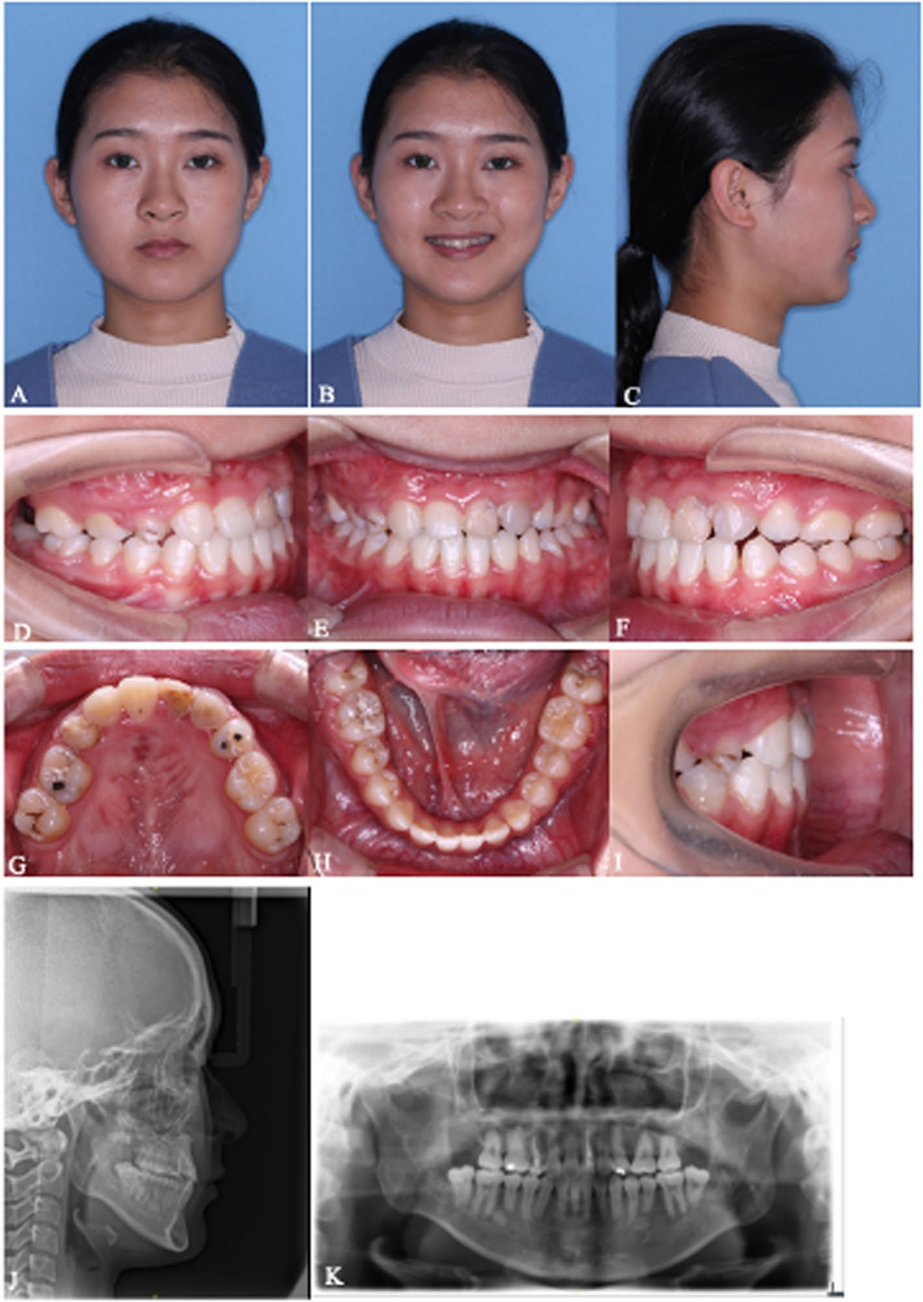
A-K After orthognathic surgery and postoperative orthodontic treatment extra- and intra-oral photographs, lateral cephalograms and panoramic of #3. The titanium plates were removed 10 months after orthognathic surgery. All appliances were removed and the final X-rays were taken 12 months after orthognathic surgery
